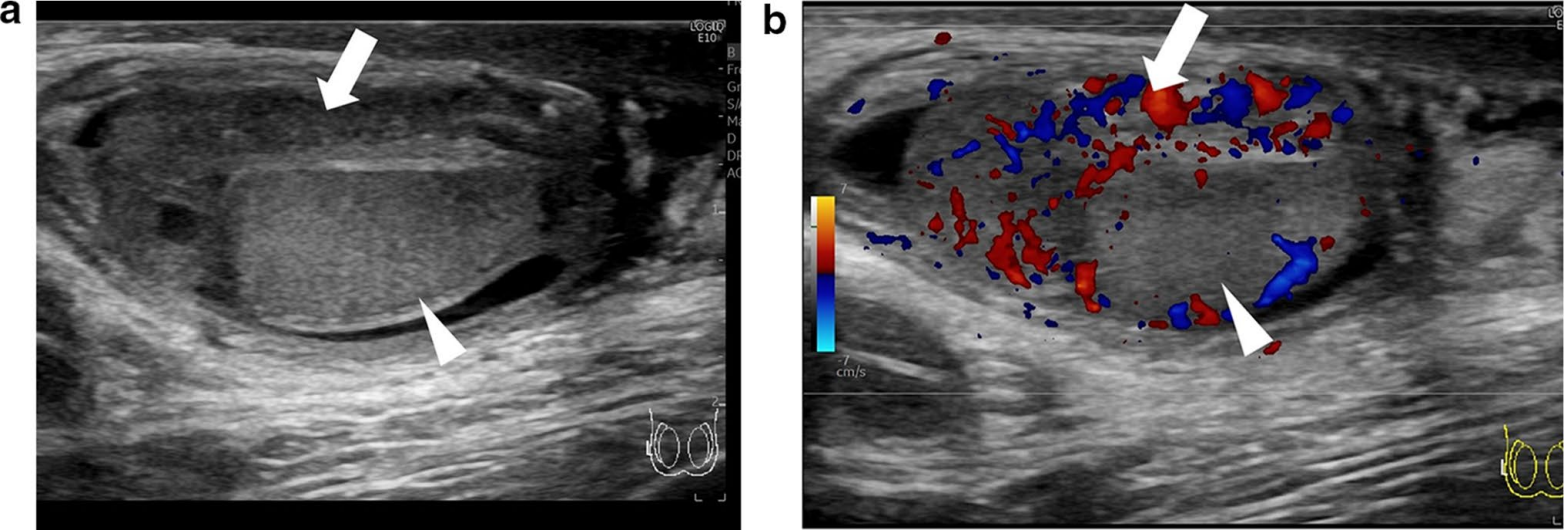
Ultrasonographic images of a 2-year-old with acute epididymitis without orchitis. a Swelling of the epididymis and edematous change in the dermis are evident. b Hypervascularity of the epididymis is evident. Acute epididymitis without orchitis was diagnosed based on these findings
